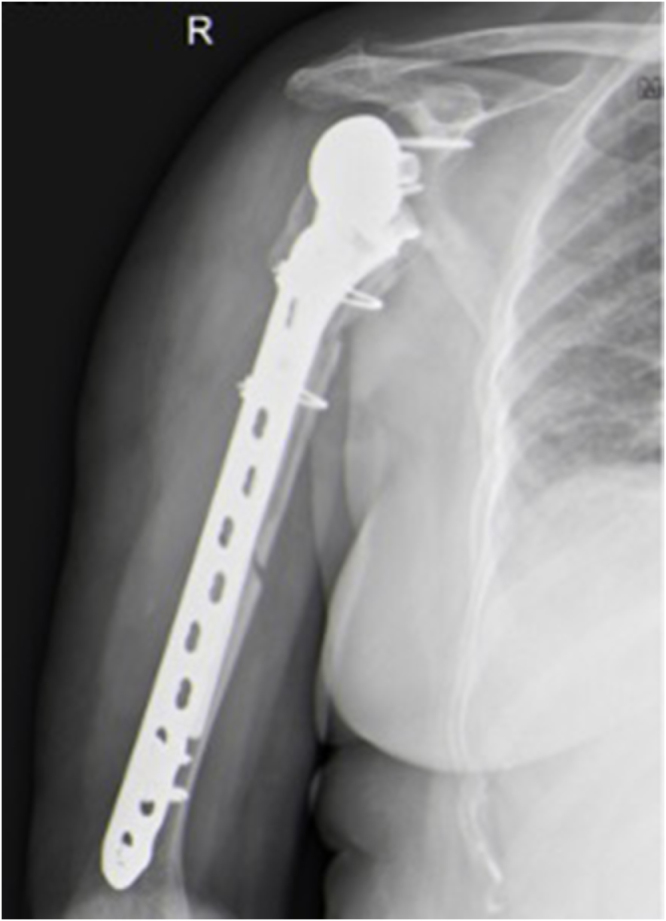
X-ray of second dislocation after first revision surgery.
